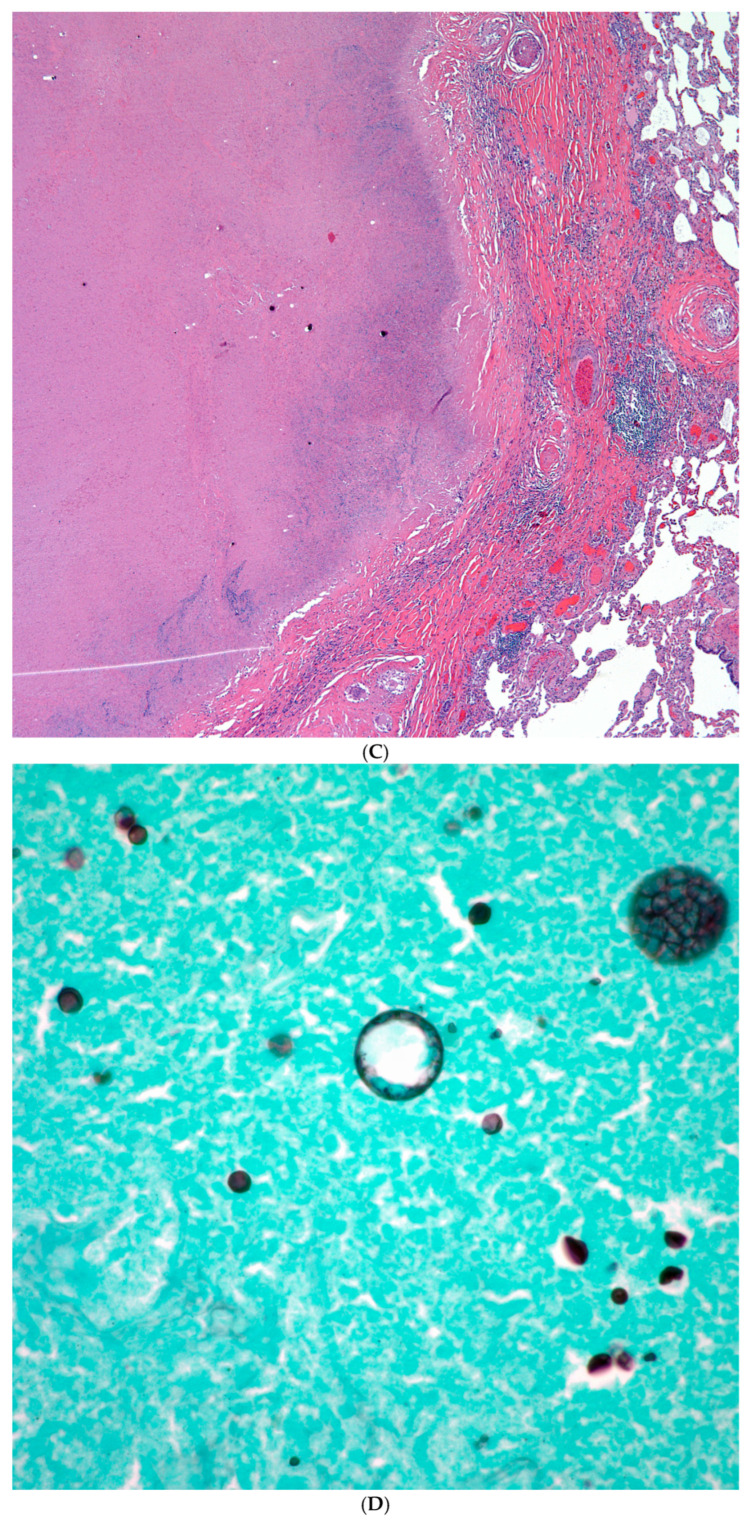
(A) CT shows left lower lobe 4 cm solid mass (arrow) abutting major fissure with adenopathy (asterisks) in left hilar and subcarinal regions mimicking lung malignancy. (B) Mass shows enhancement with administration of intravenous contrast and measures 80 Hounsfield units with lobular irregular contour on CT with lung windows. (C) Large necrotizing nodule replacing lung parenchyma. (D) GMS shows numerous large spherules containing endospores morphologically compatible with *Coccidioidomycosis* spp.
